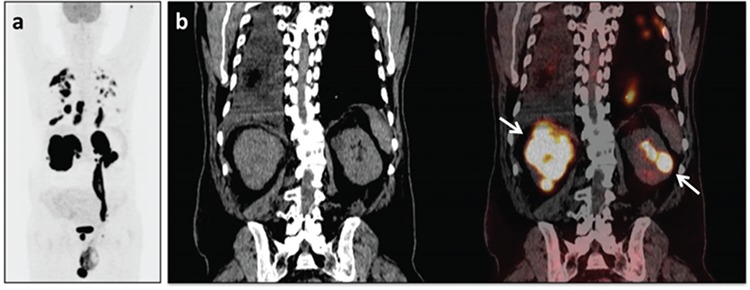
A sixty-two-year-old male patient suffering from swelling of the left testicle underwent orchiectomy and was diagnosed with diffuse large B-cell lymphoma in the testicle and spermatic cord. A) Maximum intensity projection image of the staging-intended ^18^F-FDG positron-emission tomography/computed tomography (PET/CT) scan of the case revealed multiple hyper-metabolic lymphadenopathies in the cervical, thoracic and abdominopelvic regions (SUV_max_: 34.8), hyper-metabolic lesions consistent with lymphatic/parenchymal infiltration in the lungs (SUV_max_: 16.4-45.2), pleural involvements accompanied by rib invasion on the right thorax (SUV_max_: 29.2), along with lymphoma infiltration of bilateral adrenal glands (SUV_max_: 38.7-56.5), the kidneys (SUV_max_: 40-53), the prostate gland (SUV_max_: 40.4), the right testicle (SUV_max_: 20.8) and the left testicular vein. B) Mass lesions in the kidneys are seen in selected coronal CT and fused PET/CT images.
